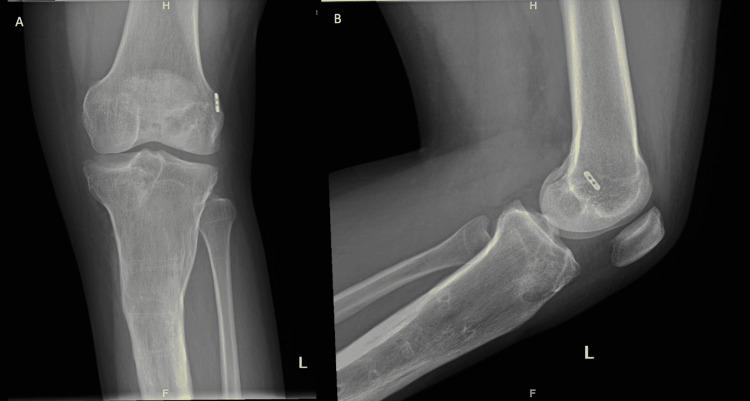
Anteroposterior and lateral X-ray of the pretibial cystic lesion 2 weeks after curettage and grafting. A) Anteroposterior X-ray of the pretibial cystic lesion 2 weeks after curettage and grafting; B) lateral X-ray of the pretibial cystic lesion 2 weeks after curettage and grafting.
